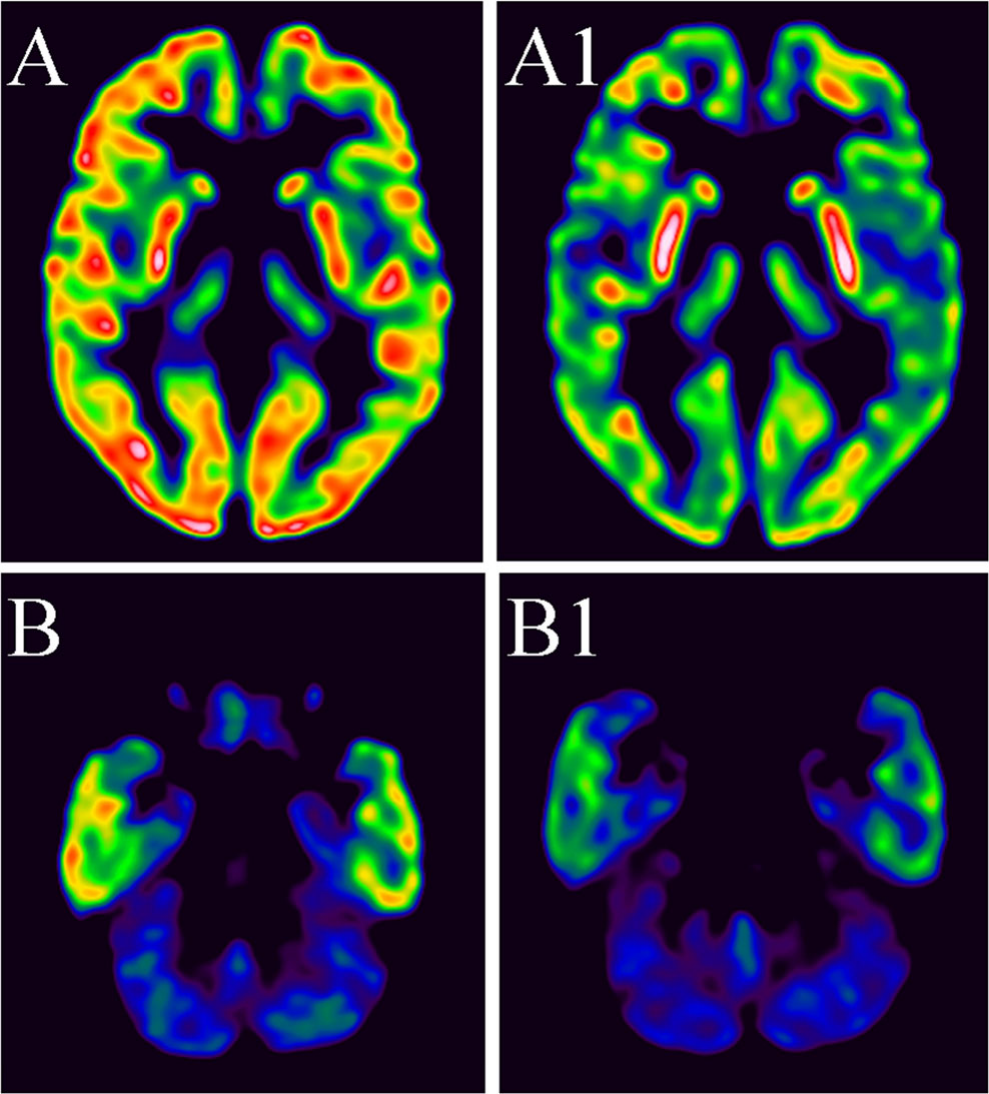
18FDG PET transaxial slices: Baseline images (ie before starting KD) at the level of basal ganglia (A) and at the level of the cerebellum (B). Decreased activity in the brain in control images at the level of basal ganglia (A1) and on the level of the cerebellum (B1)
